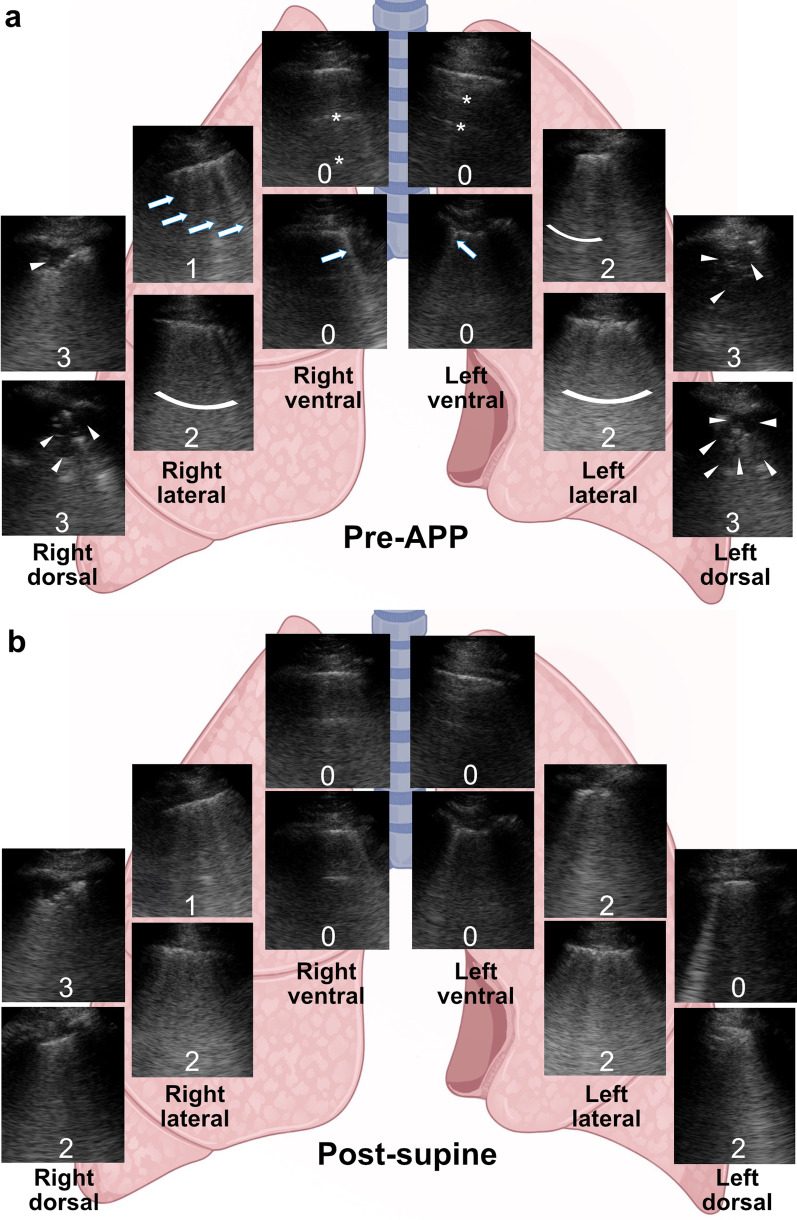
Typical changes in LUS score to APP in a patient with treatment success. a The patient’s LUS score at each lung zone before APP. Ventral zones had predominantly A-lines (asterisks) and ≤ 2 B-lines (arrows) (0 point) meaning normal aeration; lateral zones had ≥ 3 well-spaced B-lines (arrows) (1 point) and coalescent B-lines (curved bars) (2 points) suggesting moderate and severe aeration loss, respectively; and dorsal zones had irregular pleura, tissue-like pattern and subpleural consolidations (arrowheads) (3 points) suggesting complete loss of aeration. b The patient’s LUS score at each lung zone after returning to the supine position. Ventral, lateral, and upper right dorsal zones remained unchanged, while upper left dorsal zone improved from complete loss to normal aeration (3 to 0 points), and lower dorsal zones improved from complete to severe loss of aeration bilaterally (3 to 2 points). Total LUS score decreased from 19 to 14 in this patient whose first APP session lasted 5.5 h. The patient was in the supine position when LUS was performed. LUS, lung ultrasound; APP, awake prone positioning
